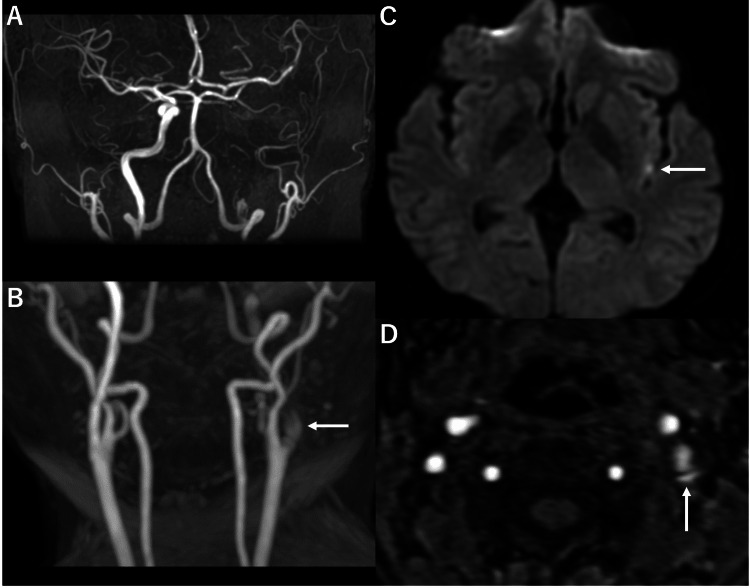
Initial magnetic resonance imaging performed at the neurosurgical clinic. A: Anterior-posterior (A-P) view of the head on magnetic resonance angiography (MRA). B: A-P view of the cervical MRA scan. The arrow indicates the occluded left internal carotid artery (ICA). C: Diffusion-weighted image. The arrow indicates asymptomatic acute infarctions. D: Source image of cervical MRA. The arrow indicates the intimal flap in the ICA, which is suspected to be a carotid artery dissection.
